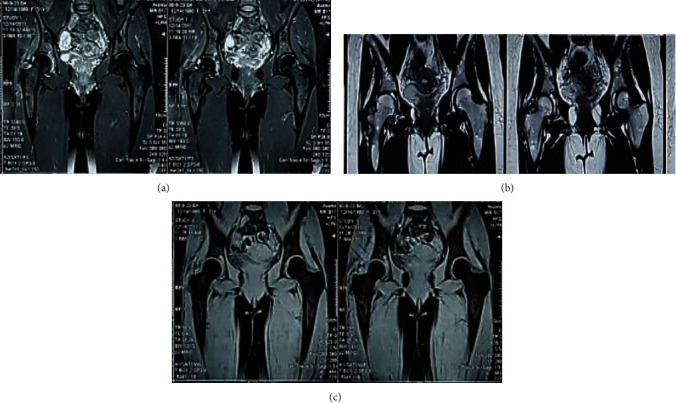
Proton density-weighted (a) MRI of the hip showing normal condition of the hip cleared from any abnormal lesion in a 6-year-follow-up visit. Postoperative 9-year follow-up T2 (b) and fat-suppressed (c) coronal MRI of the hip showing no lesion recurrence in the right hip synovial involvement.
